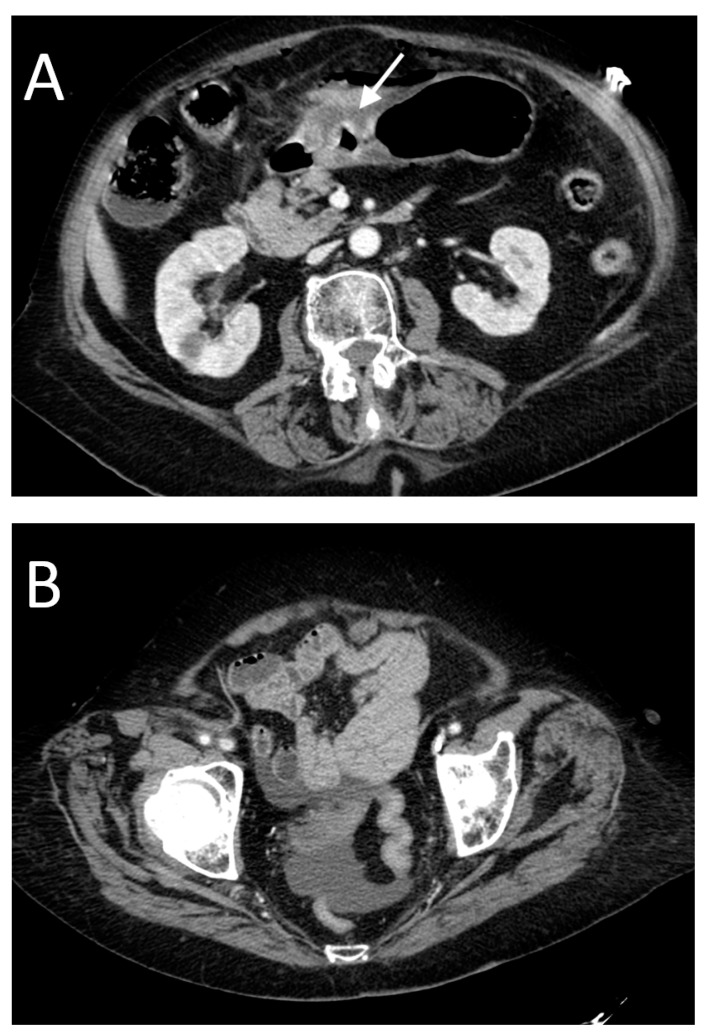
A 75-year-old female with perforation of the anterior gastric wall. In this case, CT shows focal abnormal wall thickening at the site of perforation (arrow in (A)), with the presence of a peripheral small amount of free air. There is also free fluid in the pelvis (B).
